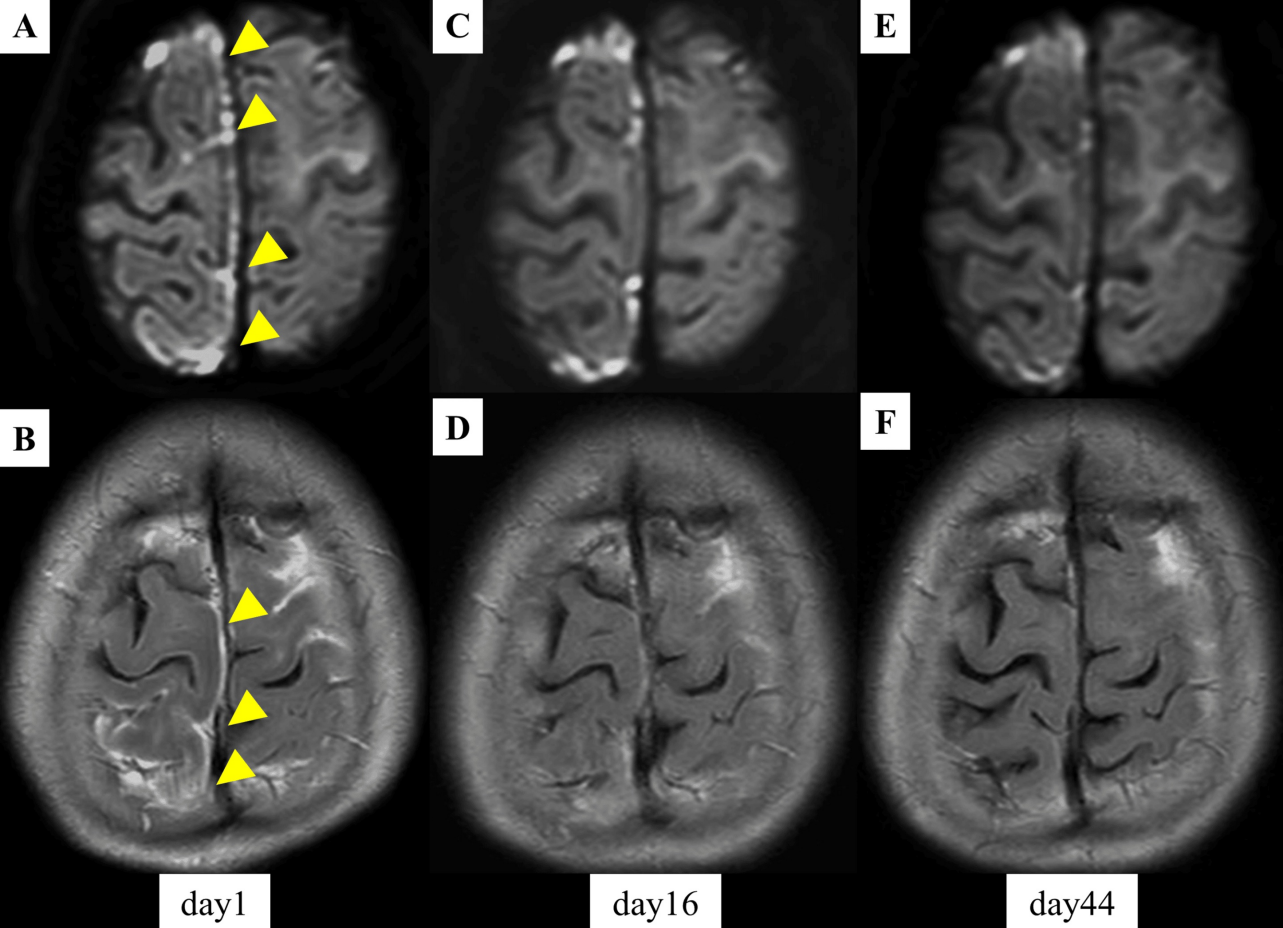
Brain MRI findings of the patient MRI findings on day 1 (A, B), day 16 (C, D), and day 44 (E, F). The upper row (A, C, E) shows diffusion-weighted imaging (DWI), and the lower row (B, D, F) shows gadolinium contrast-enhanced fluid-attenuated inversion recovery (FLAIR) images. Right-predominant cortical and brain sulcus lesions with contrast enhancement (arrow heads) gradually improved over time.
